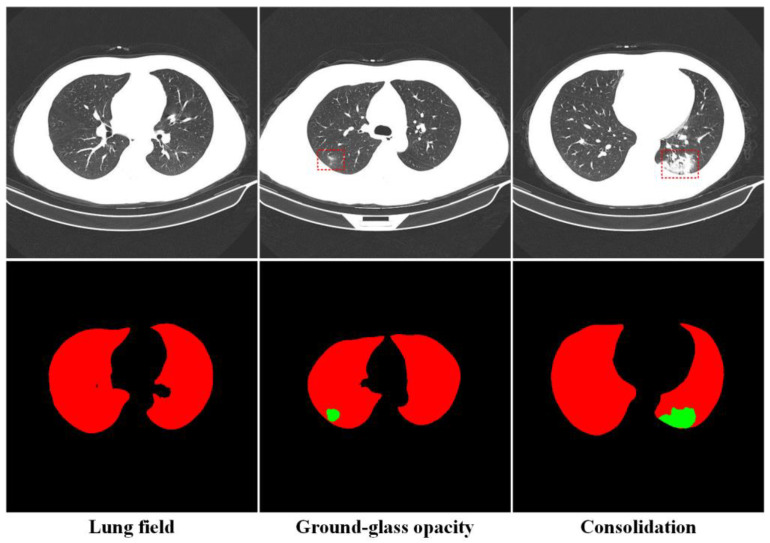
Sample images of lung field, ground-glass opacity, and consolidation in CT images.
